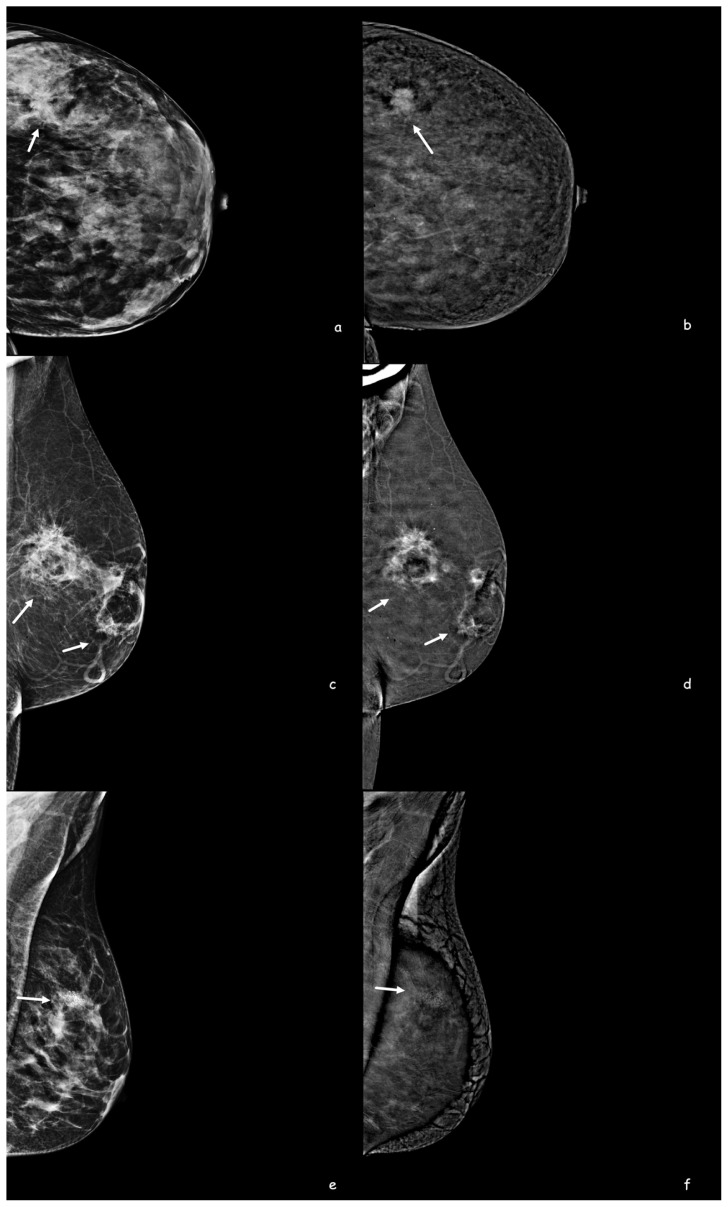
A 34-year-old woman, craniocaudal (a,) low-energy contrast-enhanced mammography (CEM) images, in the lower outer quadrant of the left breast (arrows), which is difficult to choose due to the dense breast pattern, with high-density, irregularly shaped margins that cannot be clearly seen, intense contrast enhancement in CEM subtraction (b) images. An irregularly shaped spiculated contoured mass lesion is observed (arrows). The diagnosis was invasive ductal carcinoma. A 63-year-old woman, a high-density mass in the left breast retroareolar and upper outer quadrant in MLO (c) low-energy CEM images (arrows), and an irregularly shaped spiculated contoured central fat-density mass with intense contrast enhancement on CEM subtraction (d) images lesions are observed (arrows). The diagnosis was fat necrosis. A 40-year-old woman has clustered suspicious microcalcifications in the left upper outer quadrant in MLO (e) low-energy CEM images, and there is no contrast enhancement at this level in CEM subtraction (f) images. At this level, the artifact of microcalcifications is seen. The lesion was misdiagnosed as MRI did not show contrast, and the diagnosis was ductal carcinoma in situ.
